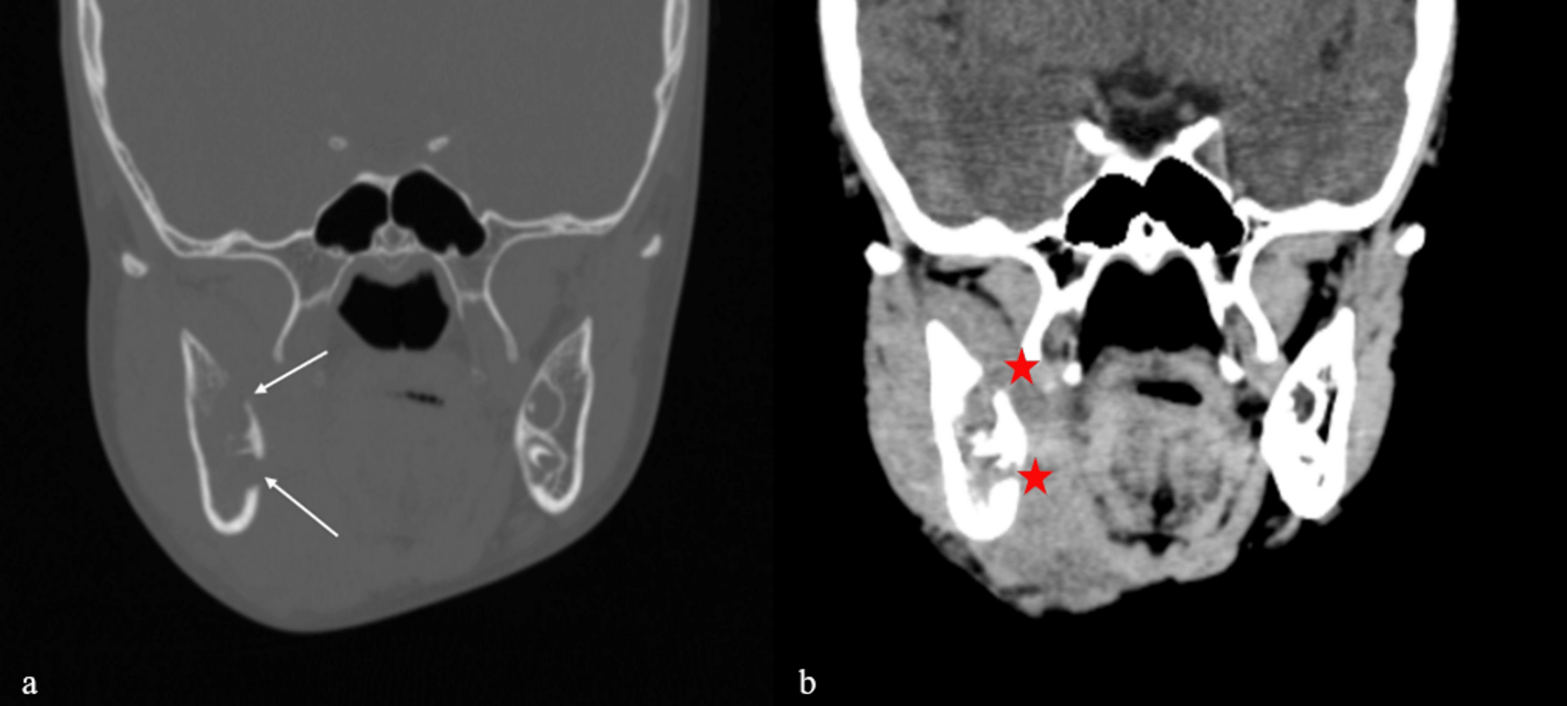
Non-contrast computed tomography (NCCT) of the face: (a) bone window demonstrating mandibular erosion with lingual cortical perforation (white arrows), (b) soft tissue window showing cortical breach with extension into the sublingual space (red stars).
